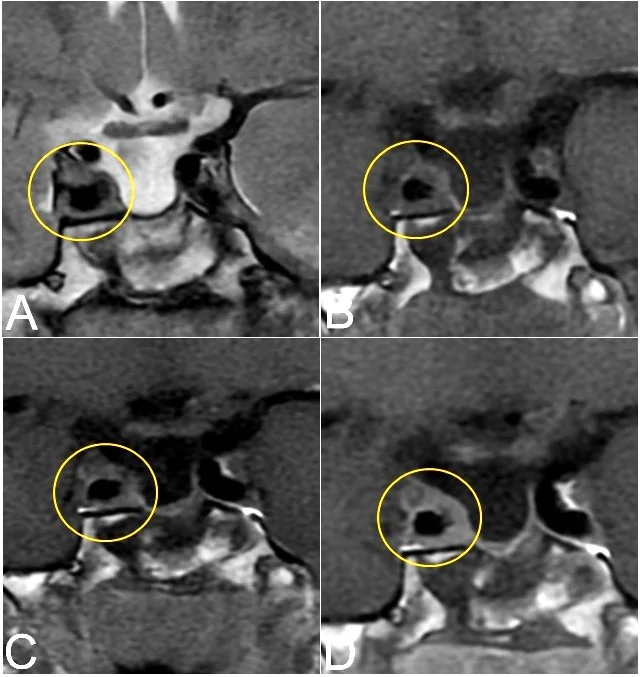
Cavernous Sinus Recurrent Adenomatous disease. Patient presented with rising serum IGF-1 (180 ng/mL, previously 96 ng/mL) and was referred for MRI (5 months since surgery). Coronal T2WI (A) and coronal T1WI (B) demonstrateabnormal signal (Circle) around the right cavernous carotid artery and within the right cavernous sinus, appearing hypointense on T2WI and isointense on T1WI relative to the white matter. On dynamic imaging, minimal heterogenous contrast uptake is seen in the early phase (C), with diffuse mild enhancement on the delayed phase (D). These observationsrepresent either residual or recurrent disease, which needs comparsion to the preoperative MRI.
